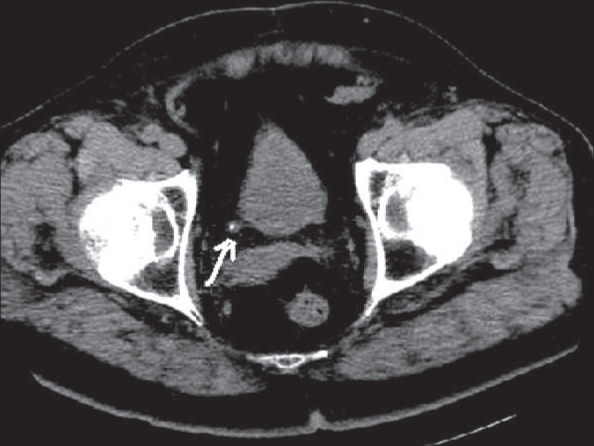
Ureteric calculus. Plain CT scan shows a ‘soft tissue rim’ sign caused by edema of the ureteric wall surrounding a calculus at the site of impaction (arrow)
